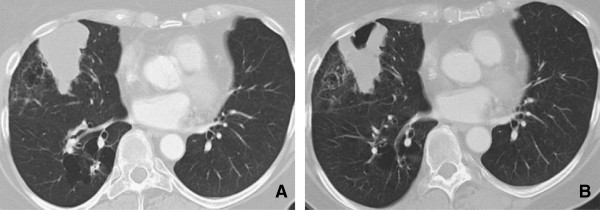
65 year old female with NSCLC. Initial CT scan shows large soft tissue mass in the RML (Figure
